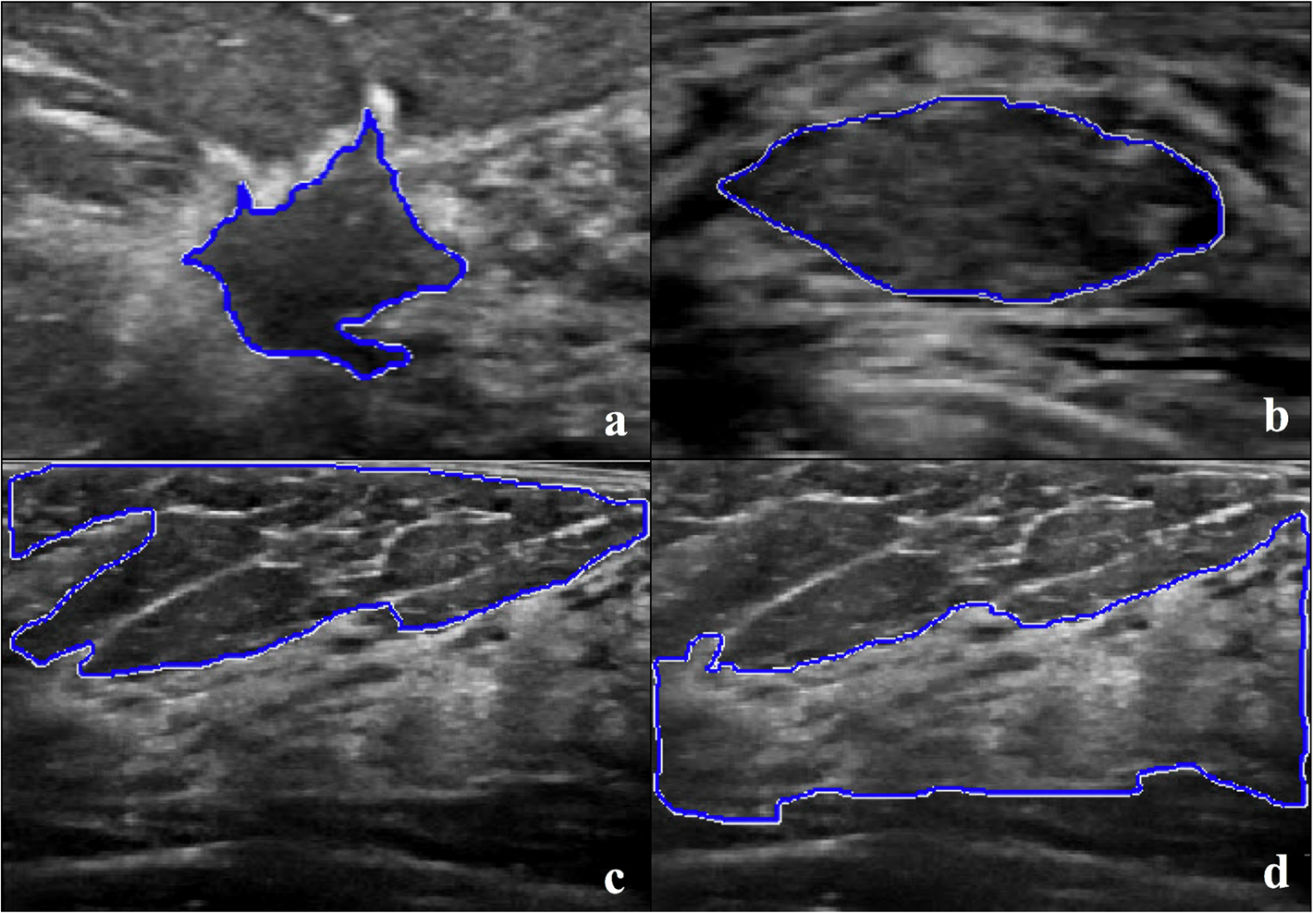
Axial images obtained with automated breast ultrasound (ABUS). A region of interest was drawn freehand marking the outer edge of the lesion or the maximal continuous area of fat or fibroglandular tissue included in the image. Invasive ductal carcinoma in a 53-year-old patient undergoing screening mammography and ABUS (a). Stable benign solid lesion after a 48-month follow-up in a 35-year-old woman undergoing routine control (b). Fatty tissue (c). Fibroglandular tissue (d)
